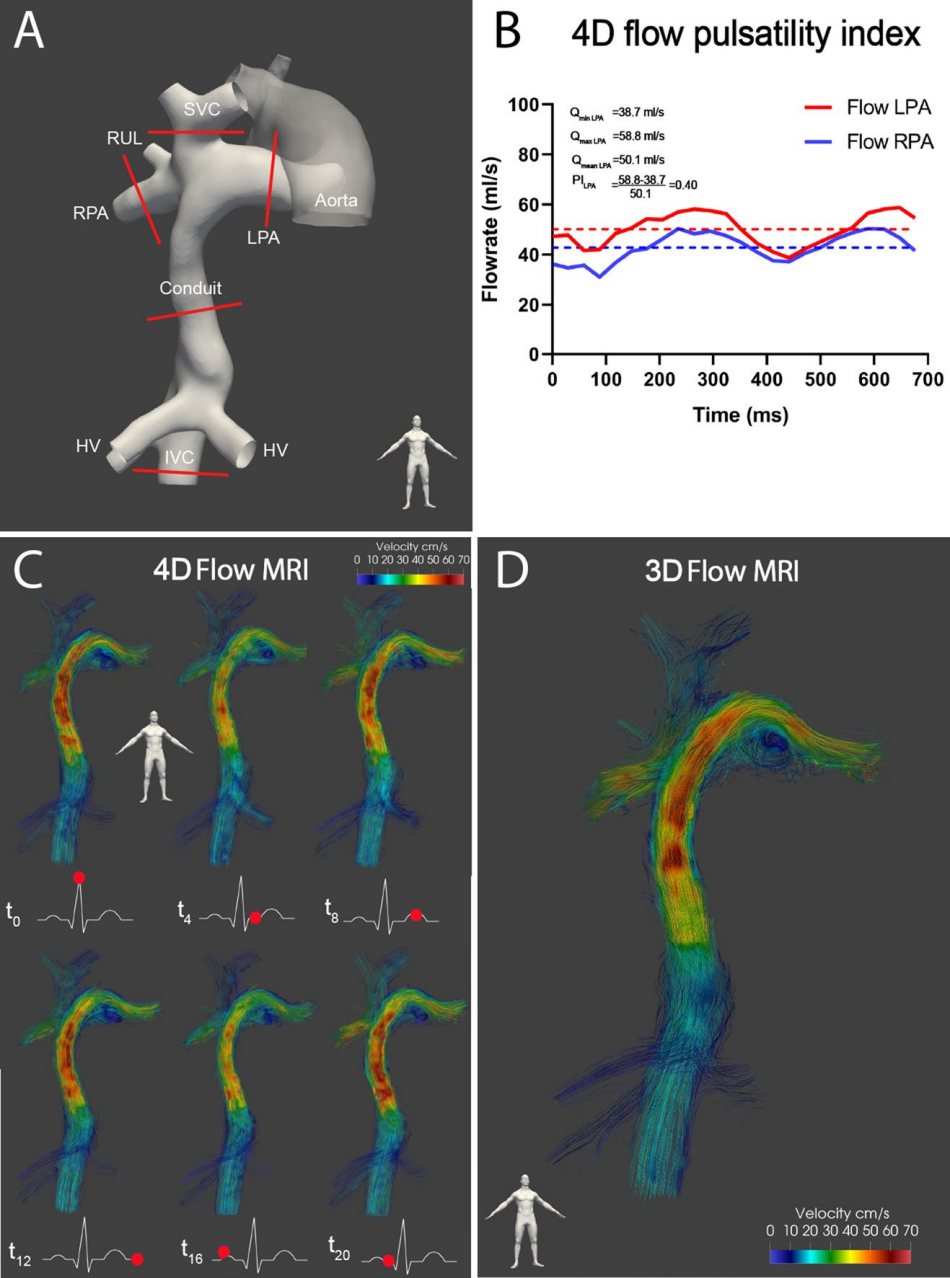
Shows a typical extracardiac conduit Fontan patient (female, 21 years old). (A) The positions of the 2D flow MRI planes are indicated in red. The aorta is shown for orientation purposes only. (B) Calculation of the 4D flow pulsatility index for the LPA is shown. (C) Streamline visualization is shown for every 4th phase in the cardiac cycle (4D flow) and for the (D) single, time-averaged phase acquired with 3D flow. Note how nearly identical flow patterns are captured with 4D and 3D flow, including the appearance of an vortex in the proximal LPA (C, D). RPA/LPA; right/left pulmonary artery, RUL; right upper lobe, IVC/SVC; inferior/superior vena cava, HV; hepatic veins, ms; millisecond, ml; milliliter, MRI; magnetic resonance imaging.
